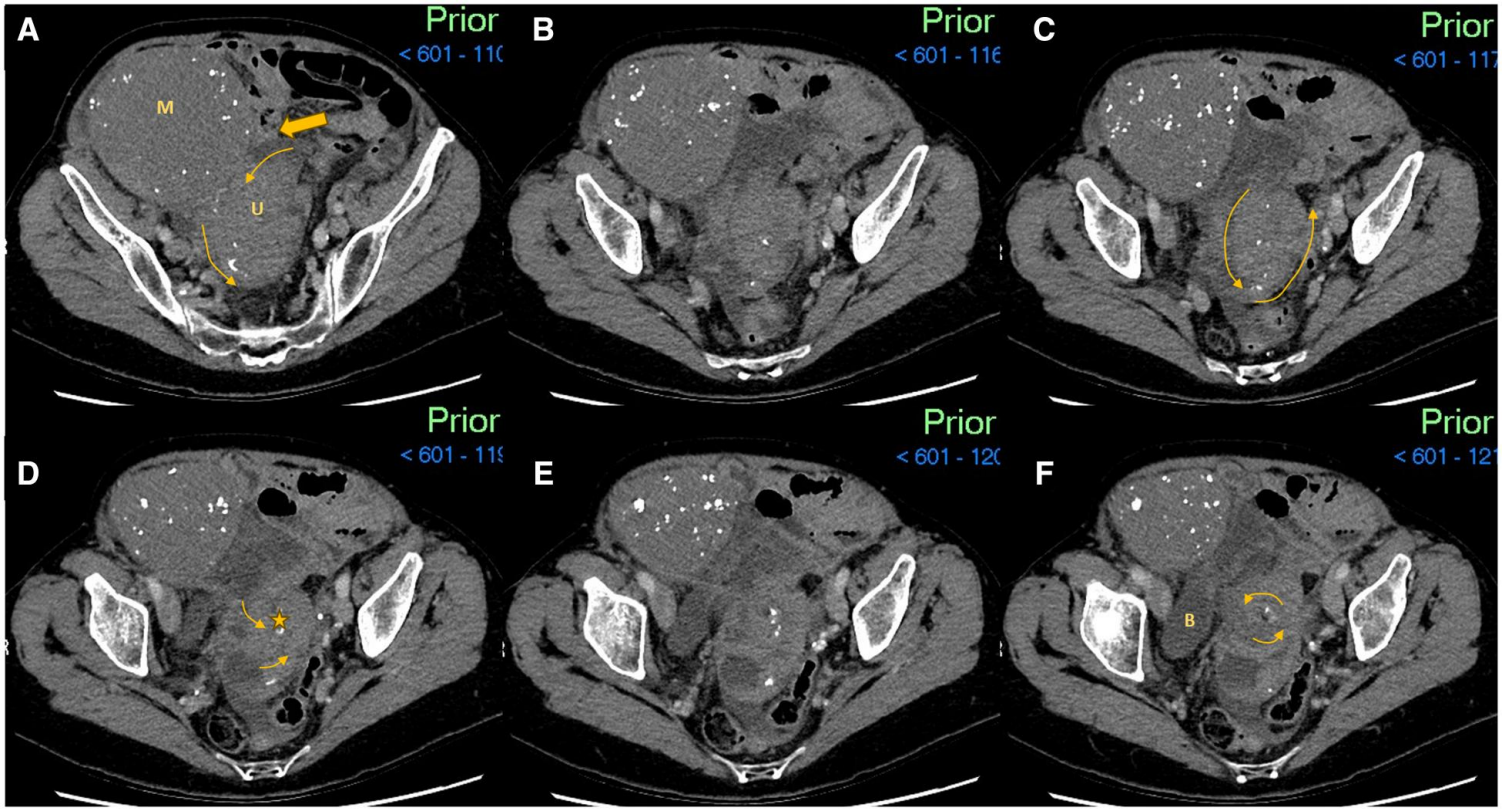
Admission CT abdomen and pelvis with contrast with selected axial images in the abdominal window setting (A-F caudally). (A) A large 17 × 11.5 × 11.3 cm fibroid mass (M) with peripheral calcifications arising from the uterine fundus. The uterus (U) is seen in the midline pelvis with the commencement of the whirling as represented by the smaller arrow heads. Upstream small bowel dilatation is not fully captured on key images, however, a point of small bowel tethering (block arrow) is noted at the uterine fundal portion. (B-F) Scrolling caudally from slices B to F the U appears to be following an anti-clockwise spiralling appearance “(whirlpool sign)”. This is best visualized on dynamic images however every effort has been made to highlight this on the following static images. The twisting/whirling is occurring around the centre point cervix highlighted by the star in image D. This is then appreciated on E and F as a hypodense centre point focus. The whirling is occurring in the axial plane and therefore sagittal and coronal CT reformats do not show the classic whirling pattern. The curved arrows (A-F) aim to depict the 540-degree rotation of the uterus around the centre point cervix, with the rotation spiralling circumference gradually getting narrower with caudal progression. The uterine wall calcifications are further supportive of the whirling appearance with calcifications being more peripheral in A and B and then more central on E and F. (E) This demonstrates speckles of calcifications entering the “vortex/epicentre,” (cervix). Additionally, the morphology of the calcifications appear comma shaped, which helps to indicate the direction of travel of the calcification and therefore alignment of the underlying uterine tissue. Abbreviations: M = uterine mass, suspected large fibroid; U = Uterus; Block arrow = point of small bowel tethering onto the uterine fundus and mass; Star (D) = cervical canal, centre point of rotation. Image slices A-F (cranial to caudal sequences).
